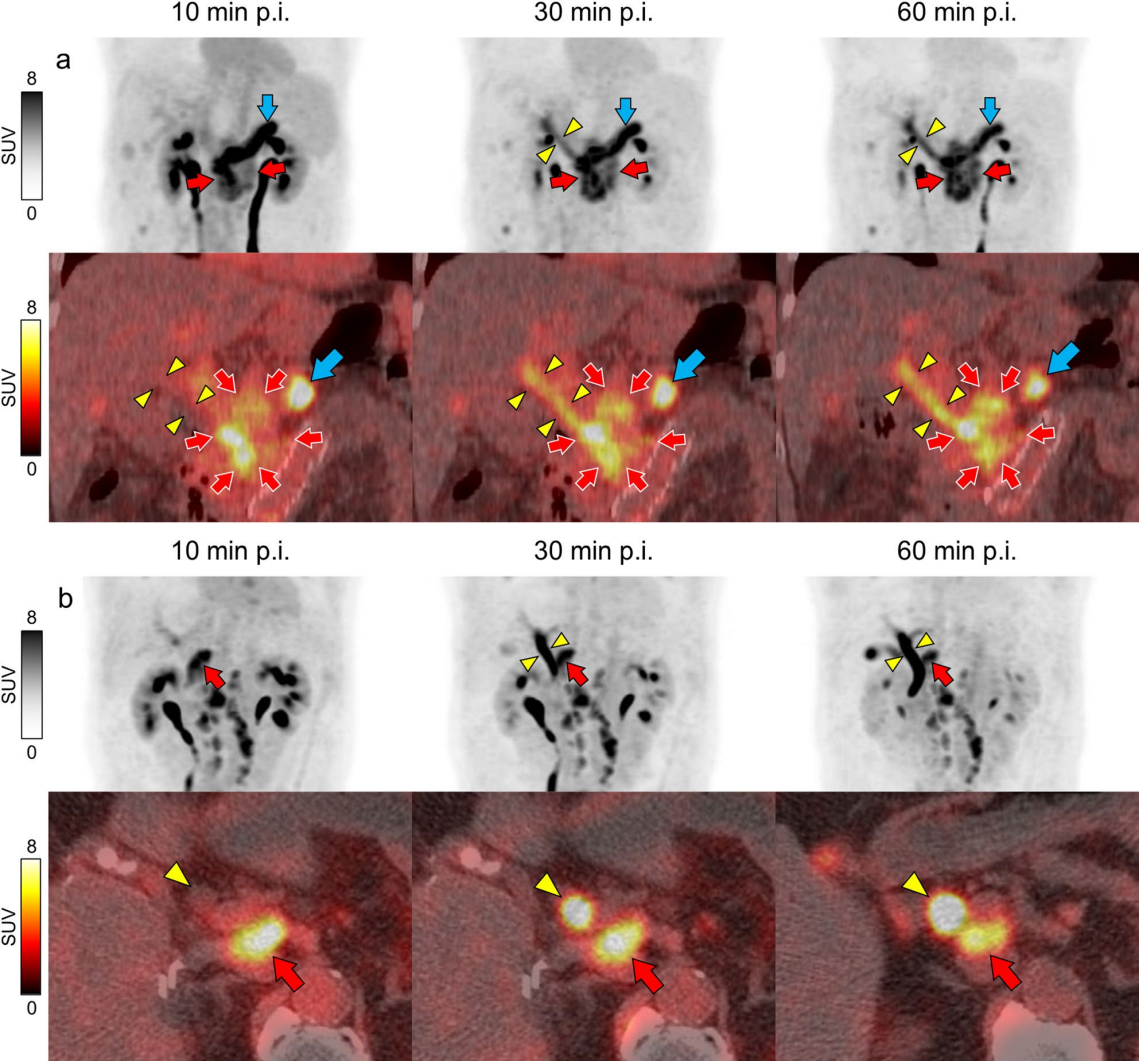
Representative cases of lesions located near the biliary system (a) MIP images and coronal [^18^F]FAPI-74 PET/CT fused images of a 62-year-old female with pancreatic head cancer. The tumor (red arrows) infiltrated the bile duct (yellow arrowheads), and uptake in the bile ducts increased over time. Increased uptake is observed in the pancreatic tail (blue arrow), suggesting fibrosis associated with chronic inflammation (b) MIP images and axial [^18^F]FAPI-74 PET/CT fused images of an 80-year-old male with para-aortic lymph node metastasis after surgery for ascending colon cancer. The metastatic lymph node (red arrow) is present on the dorsal aspect of the bile duct (yellow arrowhead), with increasing biliary tract uptake over time. Grayscales and color scales indicate standardized uptake values (SUV). MIP: Maximum intensity projection, p.i.: post injection
